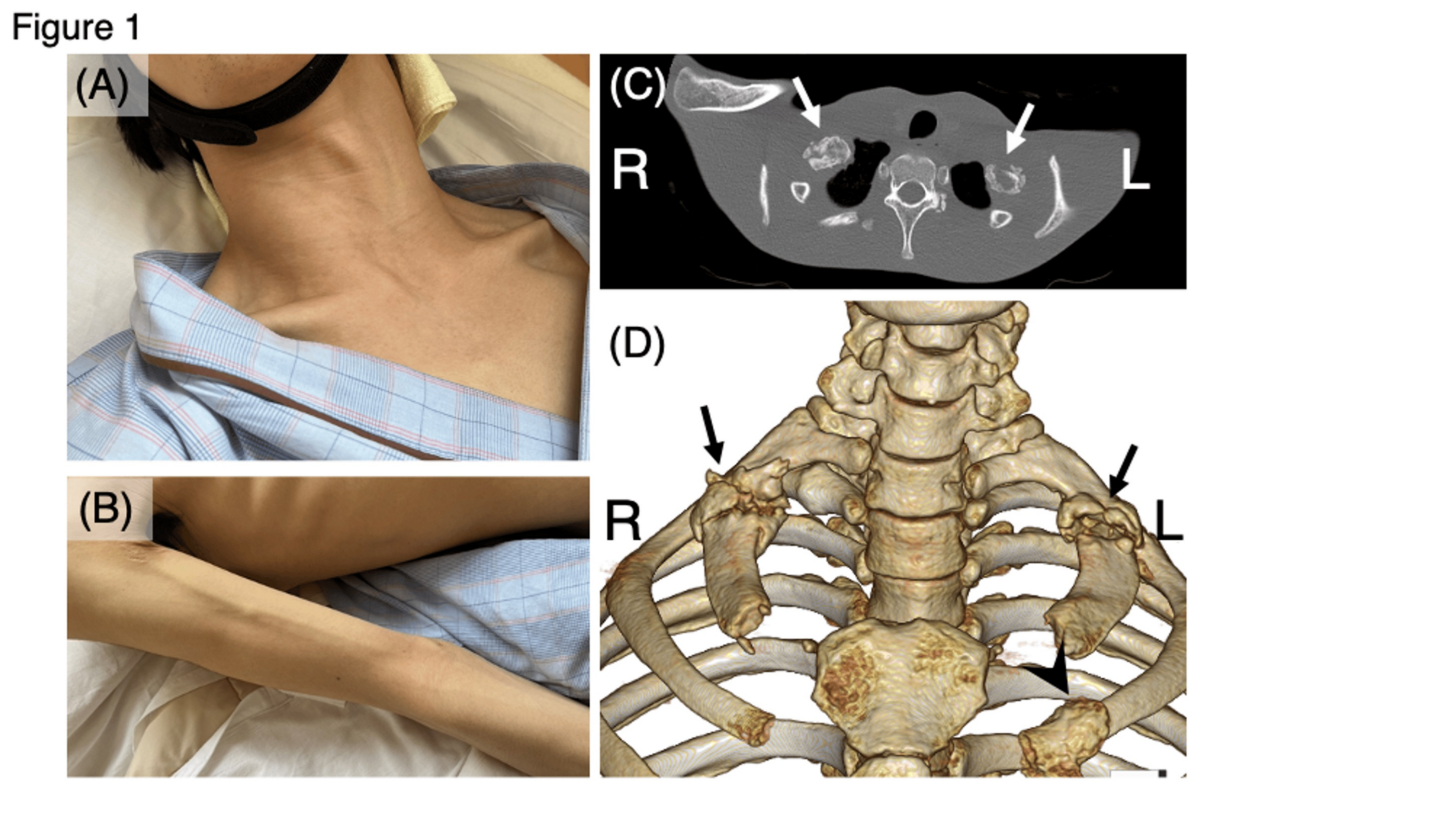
Clinical photographs and computed tomography findings (from the neck to the chest) Clinical photographs of the patient's neck (A) and right upper limb (B) show hypertrophy of the right neck muscles and atrophy of the right brachioradialis, respectively. A cross-section of the chest CT image (C) and a 3D reconstruction of the CT image from the neck to the chest (D) are shown. Bilateral scapulae and clavicles were removed from the 3D reconstructed CT image. Fractures in bilateral first ribs and callus formation (arrows) are observed. The callus is dominant on the right side. Fractures and callus formation (arrowhead) are also found in the left second rib.
